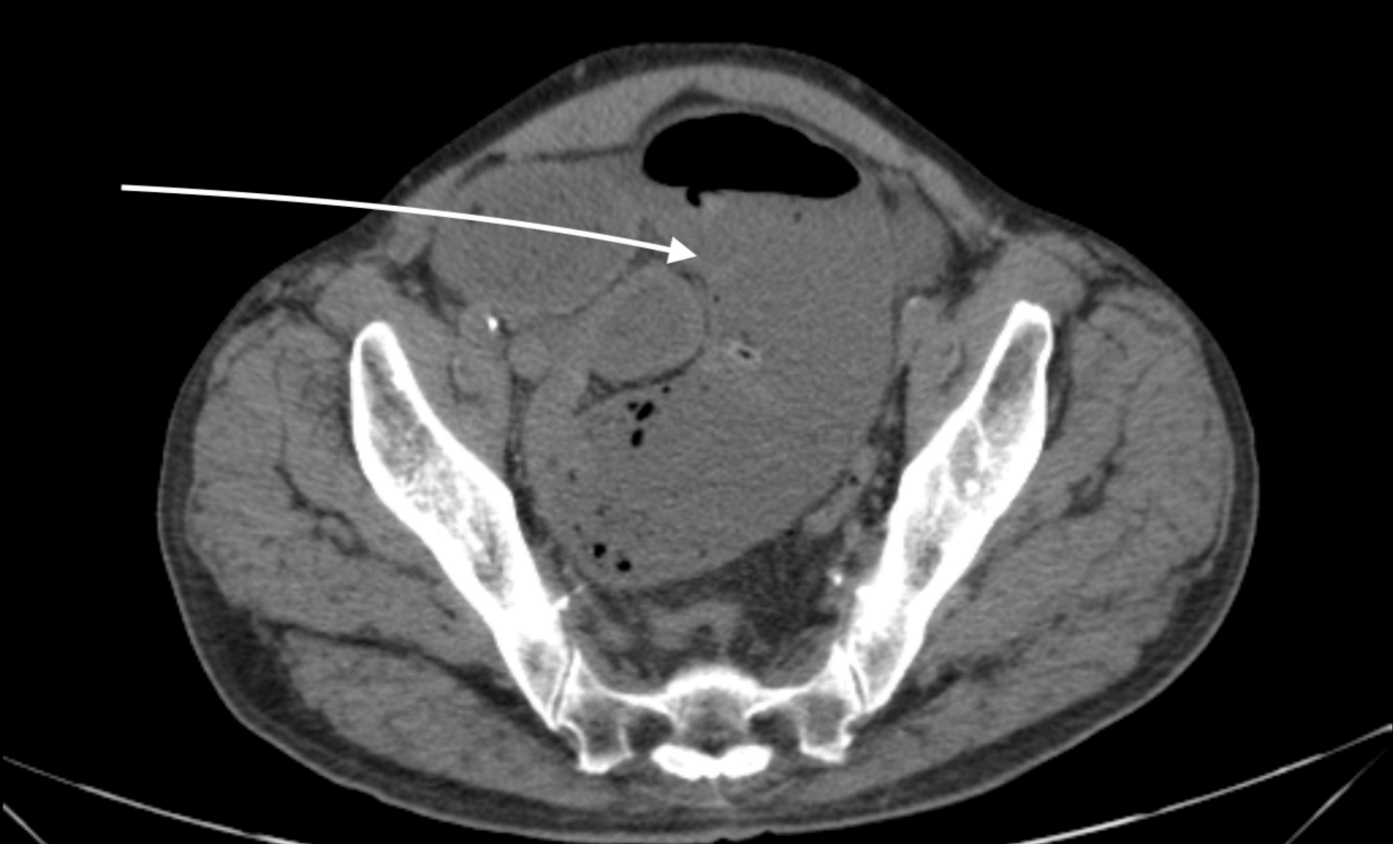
Axial view of abdominal CT scan showing diffuse small bowel distention measuring up to 5.3 cm in the pelvis, with an abrupt change in caliber in the right lower quadrant The terminal ileum collapsed.
